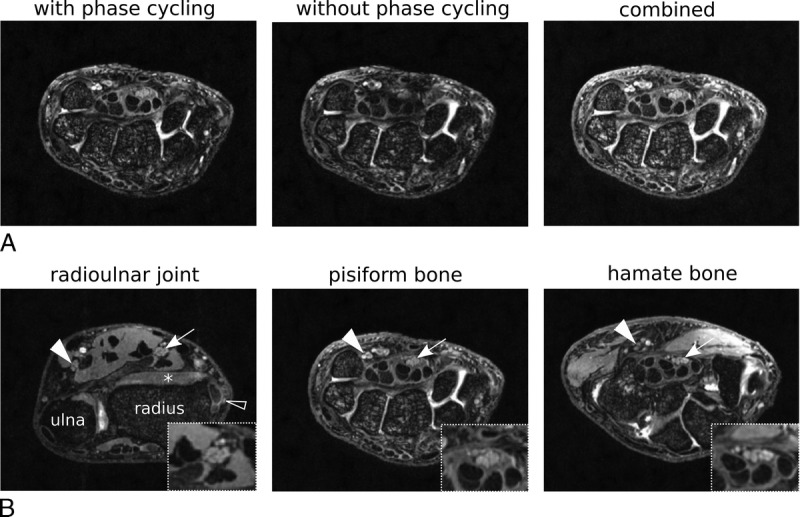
T2-weighted 3-dimensional balanced steady-state free precession (SSFP) images of the wrist acquired at 7 T. A, Reduction of banding artifacts in the SSFP images. The panels show transaxial SSFP images through the wrist acquired with and without phase cycling with clearly visible banding artifacts. The combined sum of square image largely eliminates banding artifacts, despite the severity in the two original images. B, Combined SSFP images. Panels show the median nerve (arrow) at the level of the radioulnar joint (left), pisiform bone (middle), and hook of hamate (right). The high spatial resolution of the 7 T images allows high visibility of morphologic details such as the differentiation of fascicles within the median nerve (zoomed insets). The 7 T morphologic sequences also allow visualization of the ulnar nerve (filled arrowhead) and the superficial radial nerve (empty arrowhead) at the wrist. The peripheral nerves are isointense with muscle (asterisk).
